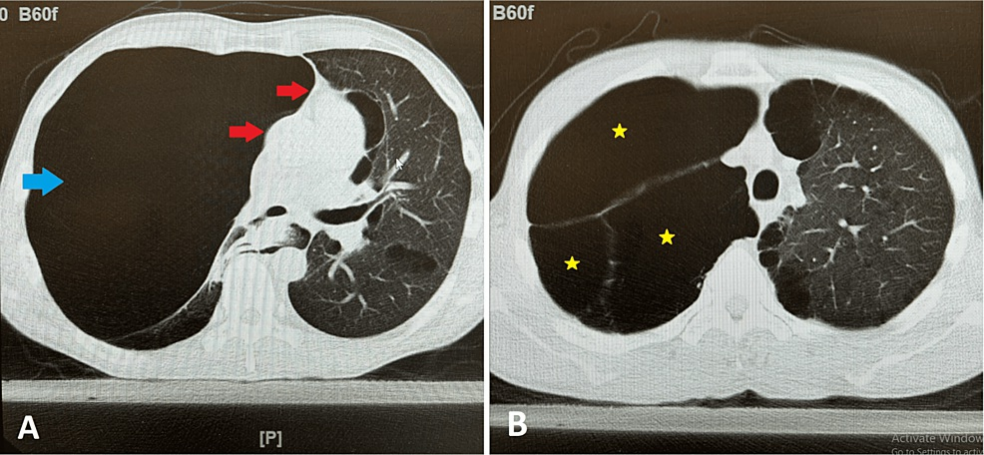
Non-contrast CT of the chest of the same 50-year-old male patient (A) Imaging shows a near-entire right lung involvement (blue ➨) and a mediastinal shift to the left (red ➨). (B) The right lung demonstrates multiple large bullous emphysema adjacent to one another (yellow ★). CT: computed tomography
